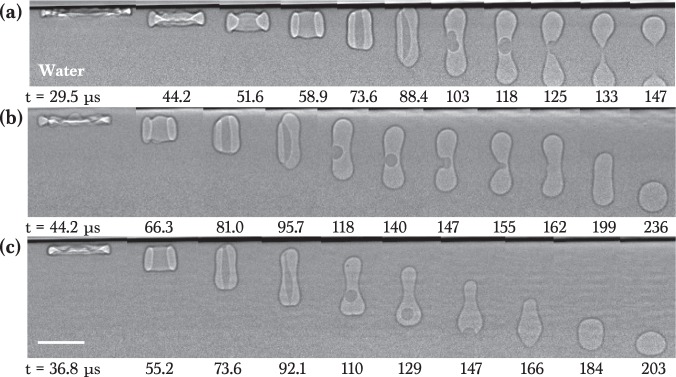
Sequential X-ray images of evolution of the entrapped bubble for water. (a) The axisymmetric coalescence forms the bubble split into two bubbles. (b) The one-sided coalescence results in one bubble. (c) The bottom-sided coalescence of the daughter droplet in a peanut-like bubble also results in one bubble. The scale bar is 100 *μ*m long.
